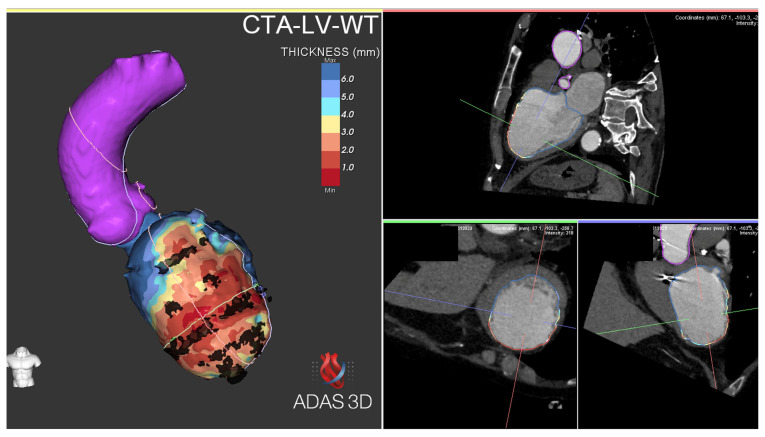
MDCT imagery from an ischemic patient post-processed with a dedicated software (ADAS 3D). A Three-dimensional reconstruction of the aorta (pink) and left ventricle with lipomatous metaplasia (transparent black) and wall thickness analysis. LV wall thickness is shown as a color map (from blue > 6 mm to red < 1 mm). LV, left ventricle; MDCT, multidetector CT.
